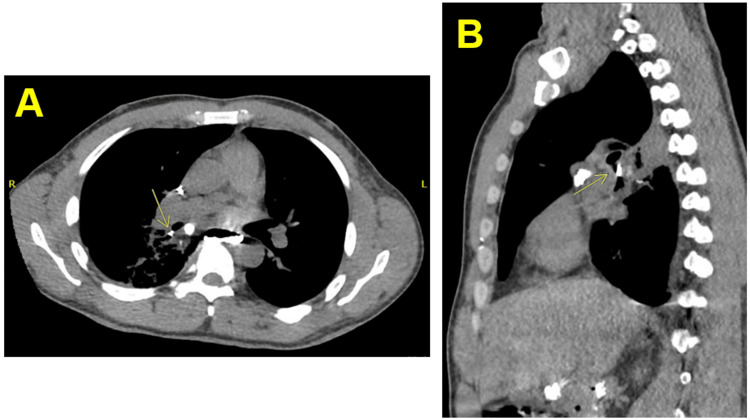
Axial (A) and sagittal (B) CT of the abdomen and pelvis demonstrating oral contrast in the right mainstem and adjacent right lower lobe bronchus compatible with BEF (yellow arrows), right hilar fullness with bronchial narrowing and wall thickening, and increased opacity along the posterior medial right lower lobe with pleural thickening. BEF: Bronchoesophageal fistula
